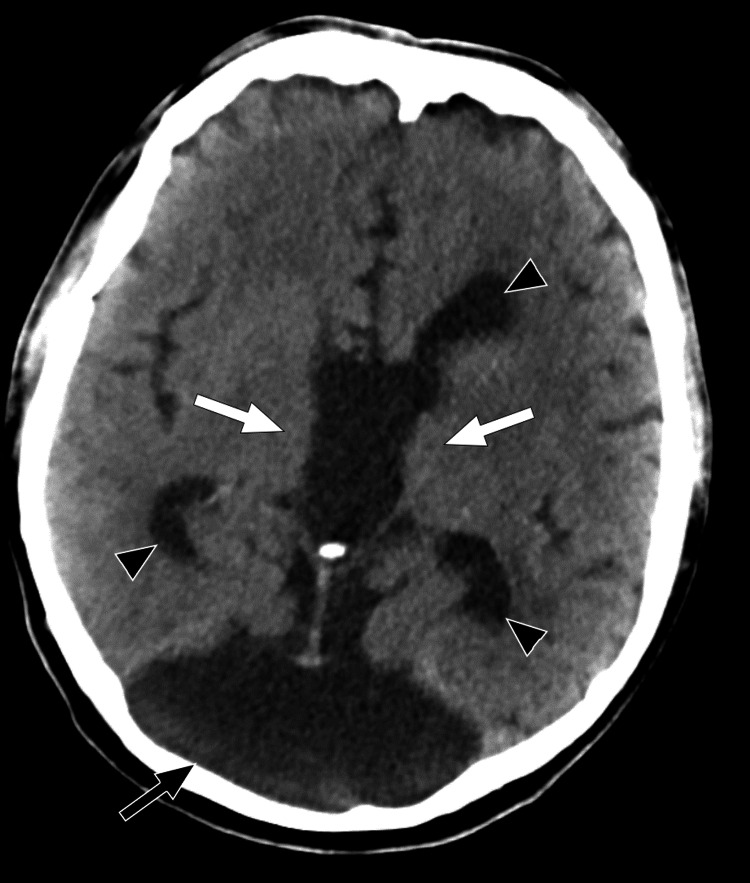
Axial CT of the brain at the level of the thalamus reveals an absence of thalamic fusion (white arrows). The third ventricular component of the monoventricle, along with the frontal horns and trigone of the lateral ventricular components (black arrowheads), can be seen at this level. Additionally, the posterior fossa cyst of the Dandy-Walker malformation (black arrow) is partially visible.
